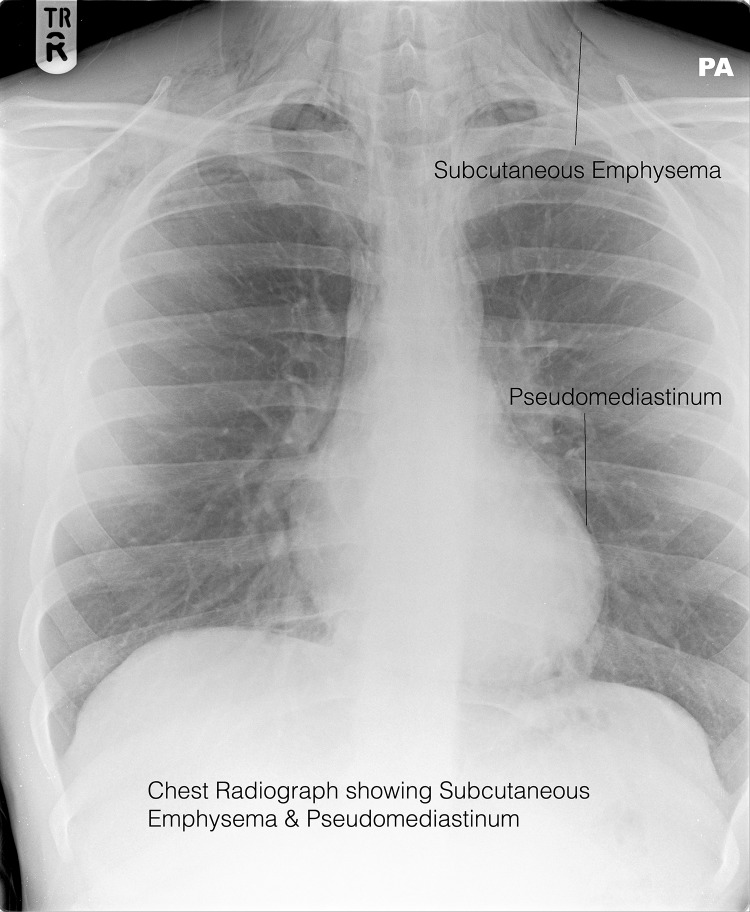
Chest radiograph demonstrating pneumomediastinum and extensive cervical subcutaneous emphysema
